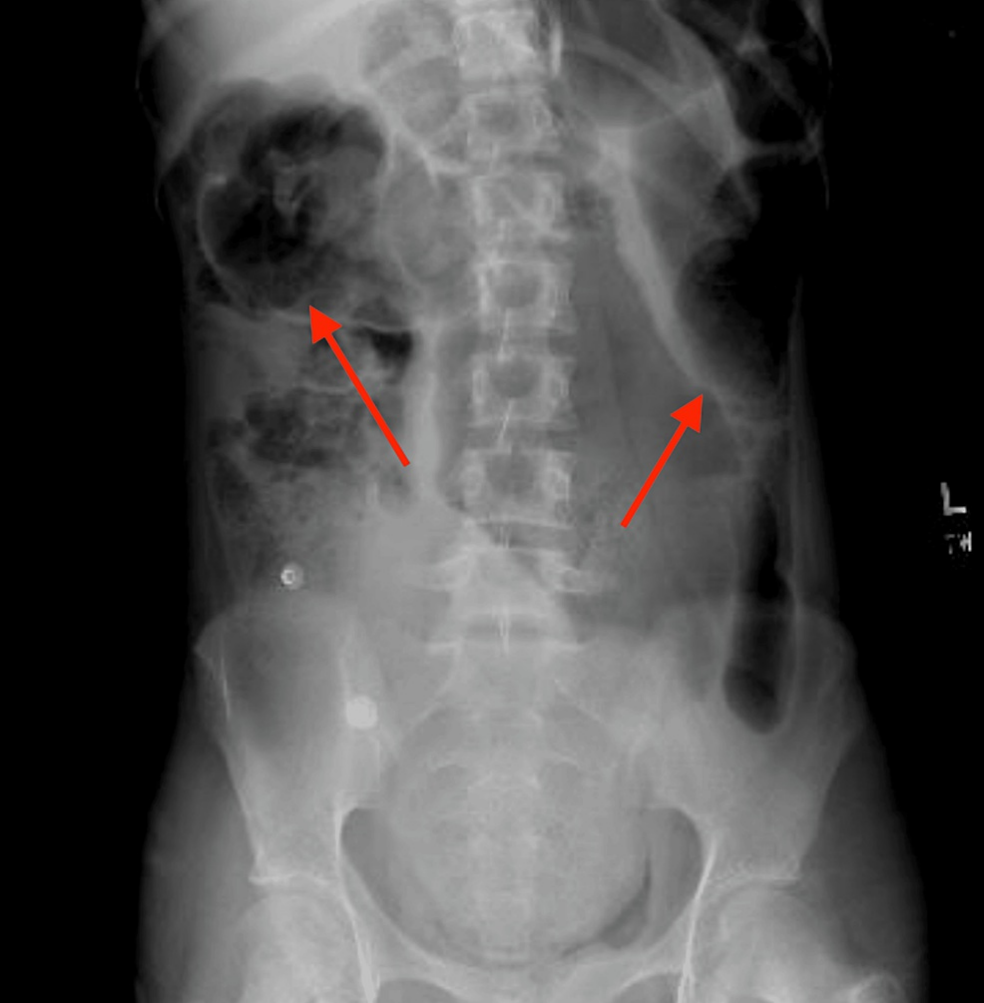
Radiograph indicating dilated loops of bowel consistent with a possible obstruction.
